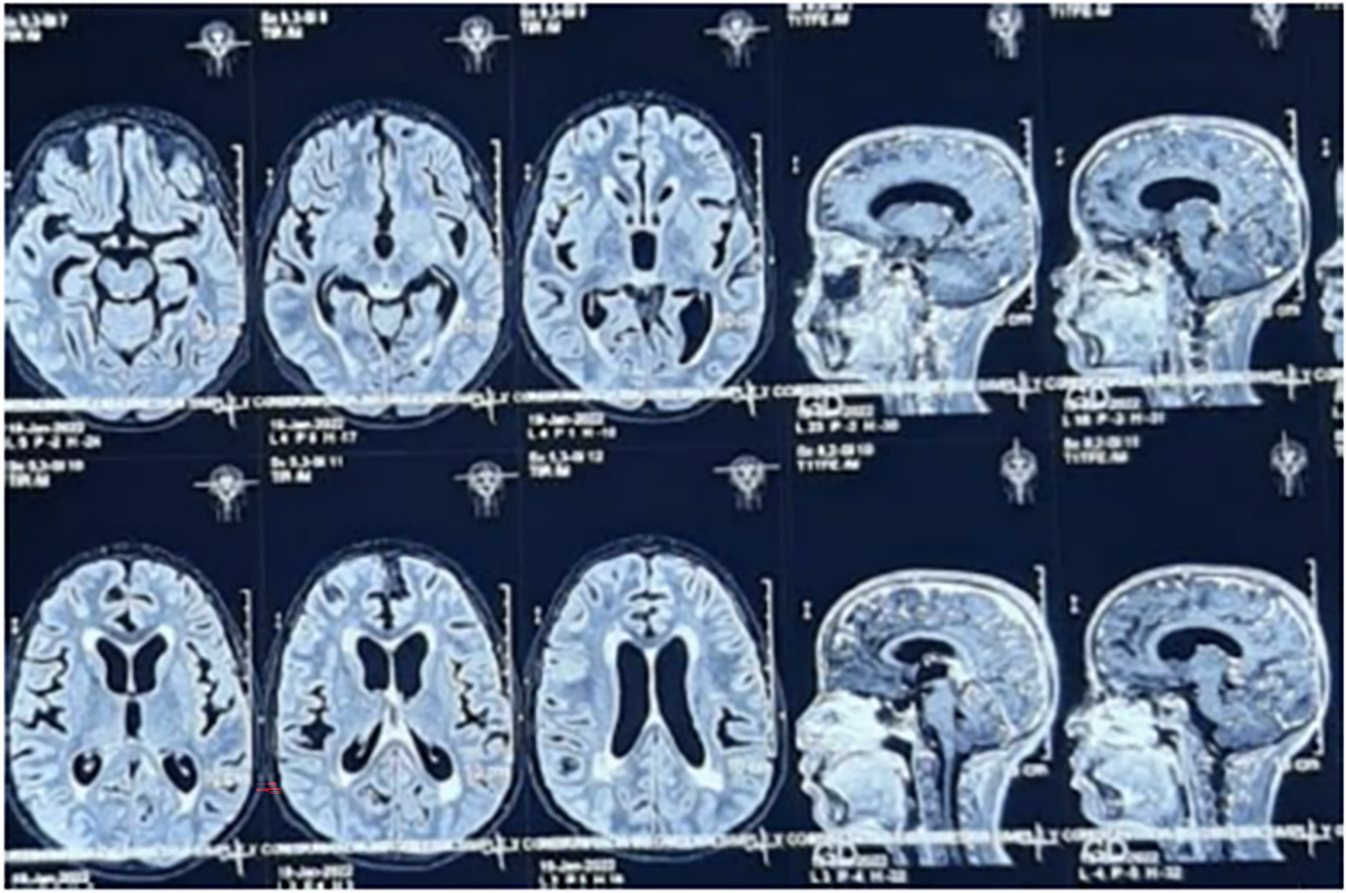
MRI without contrast shows evidence on T2-weighted sequences of abnormal contrast enhancement of the cerebral and cerebellar leptomeninges, cortical and central cerebral atrophy, and ventricular system dilation.
